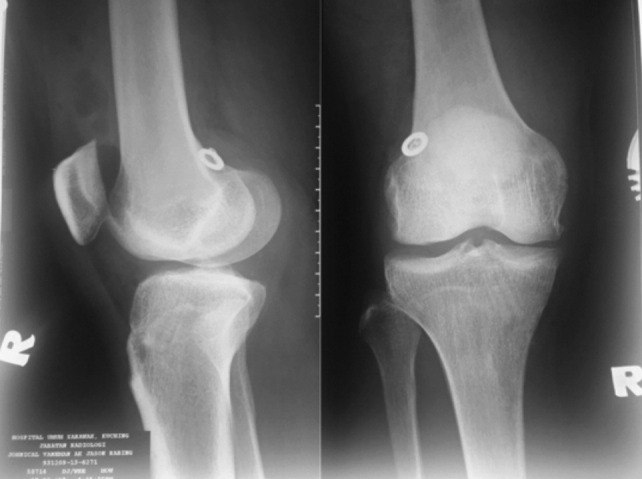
Post-operative radiographs of right knee showing placement of the endobutton and washer over the lateral femoral wall.
